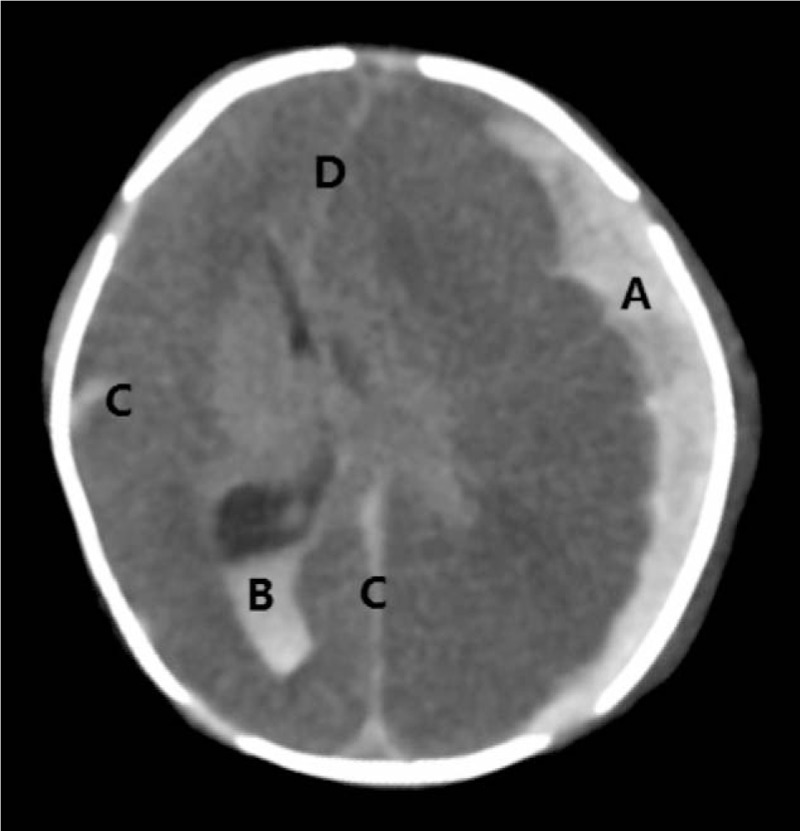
The head CT scan. It was shown that a severe subdural hematoma (A) causing midline shift (D), bilateral intraventricular hemorrhage (B), and subarachnoid hemorrhage (C).
